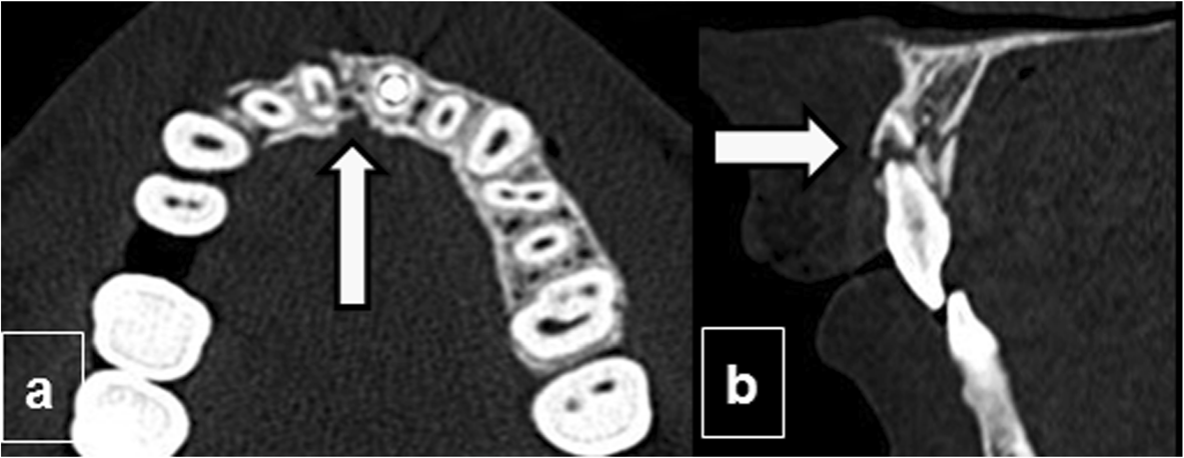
Alveolar process fracture. Axial (a) and sagittal (b) unenhanced CT images show a fracture of the upper alveolar ridge with associated dental root fracture (arrows)
